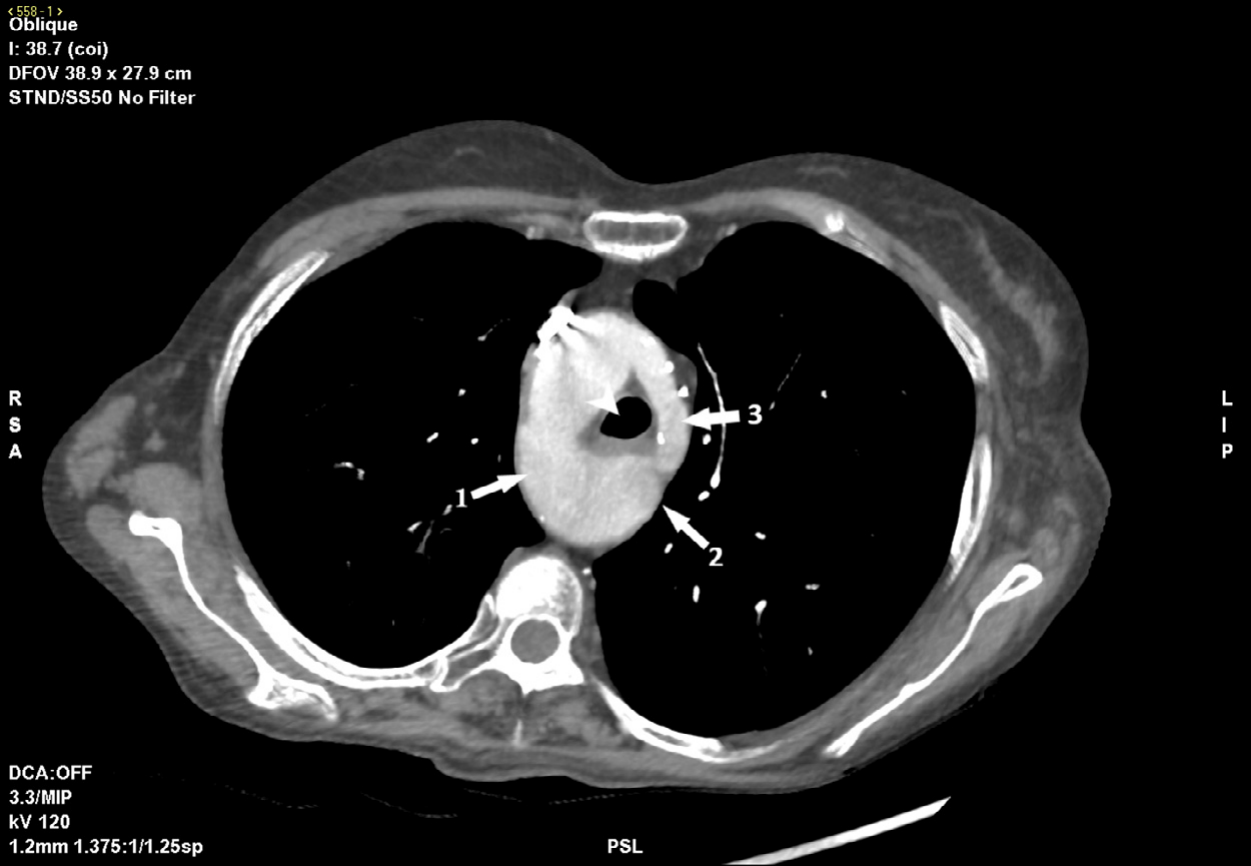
Oblique-axial MIP view of angiographic-CT-scan, showing evidence of a vascular ring surrounding the middle tract of the trachea and esophagus (white arrowhead), determined by the right aortic arch (white arrow nº1), the aortic diverticulum (white arrow nº2) and the residual left arch (white arrow nº3).
